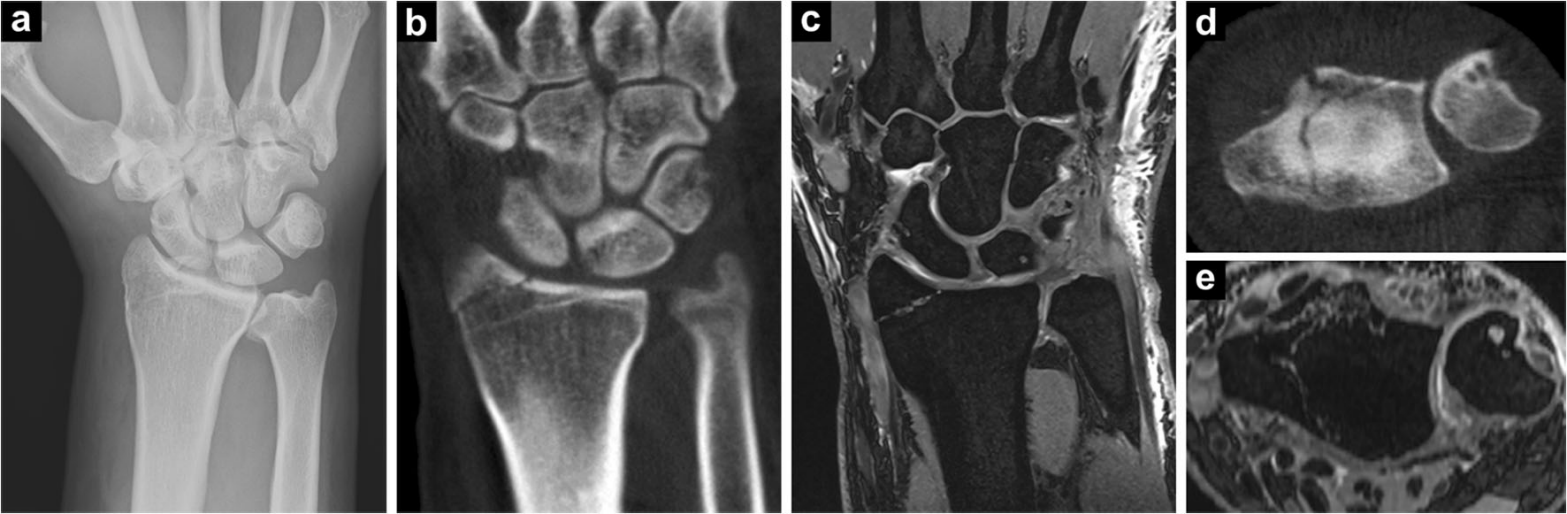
While unloading a truck, a 51-year-old man fell from approximately 2 m height onto his head and right hip. Also reporting right wrist pain, a simple non-displaced fracture of the radial styloid was surmised after radiography (a). Coronal reformatting of subsequent cone-beam CT (b) confirmed the suspected injury, whereas axial view (d) disclosed additional fracture lines in the articular surface of the distal radius. Coronal and axial multiplanar reconstructions of 3-D dual-echo steady-state MRI (c, e) validated cone-beam CT findings. Treatment was upgraded from immobilization to surgery
